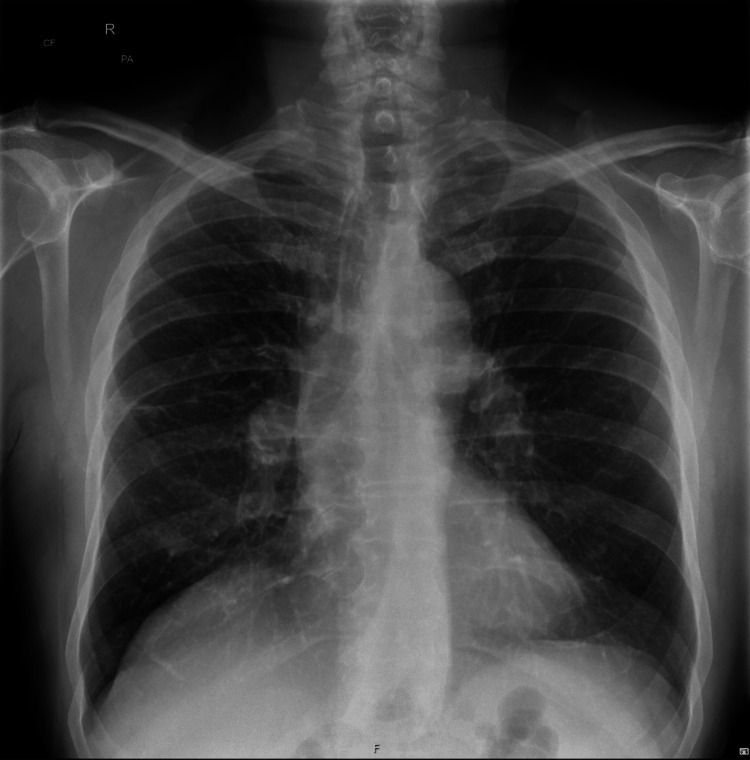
Chest x-ray (CXR) eight months later showing complete resolution of the cavitating lesion
